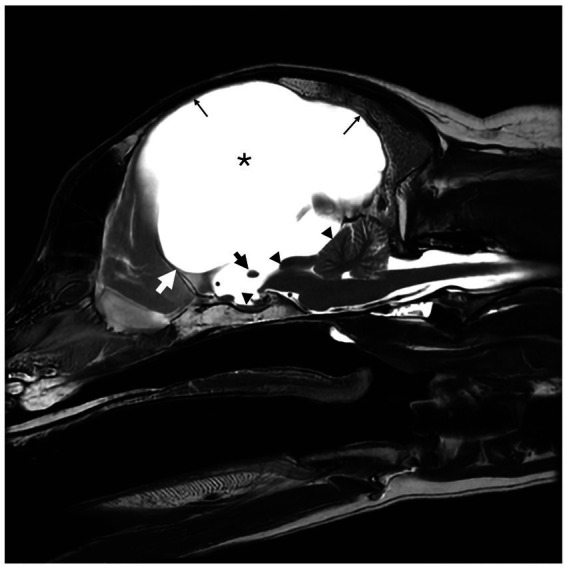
T2-weighted (T2W) sagittal magnetic resonance image (MRI) of a 6-year-old male neutered golden retriever. Marked hydrocephalus with thinning of the cerebrum secondary to ventriculomegaly is noted by (*). The small black arrow notes the markedly thinned interthalamic adhesion. The small white arrow delineates the rostral aspect of the third ventricle, at the level of the lamina terminalis. The corpus callosum is absent. The mesencephalic aqueduct is markedly narrowed with secondary rostral flattening of the cerebellum and compression of the pituitary gland (small black triangles). There is diffuse thinning of the cerebral cortex (thin black arrow).
